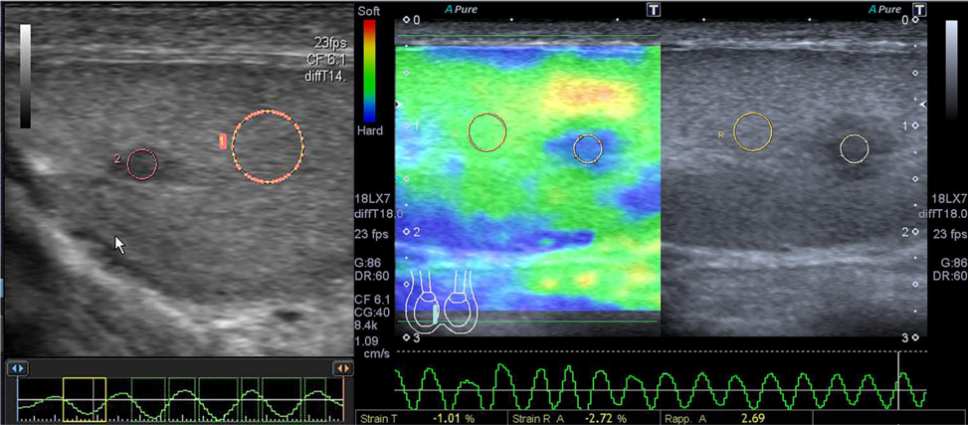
Solid nodule (7 mm) with high SR (2.69) discovered during ultrasound scan in a 36 years old patient with primary infertility.
